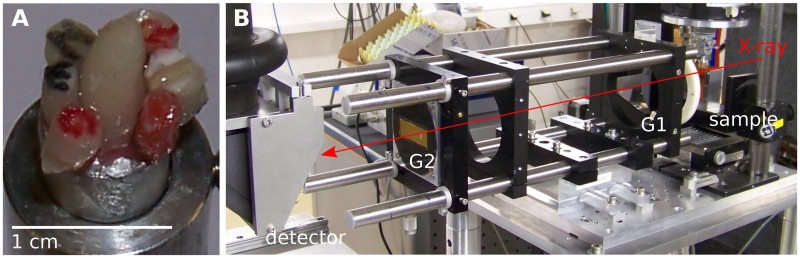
Experimental setup. A) The tooth sections imaged in this study. B) The x-ray grating interferometer at the imaging beamline ID19 of the European Synchrotron Radiation Facility.
